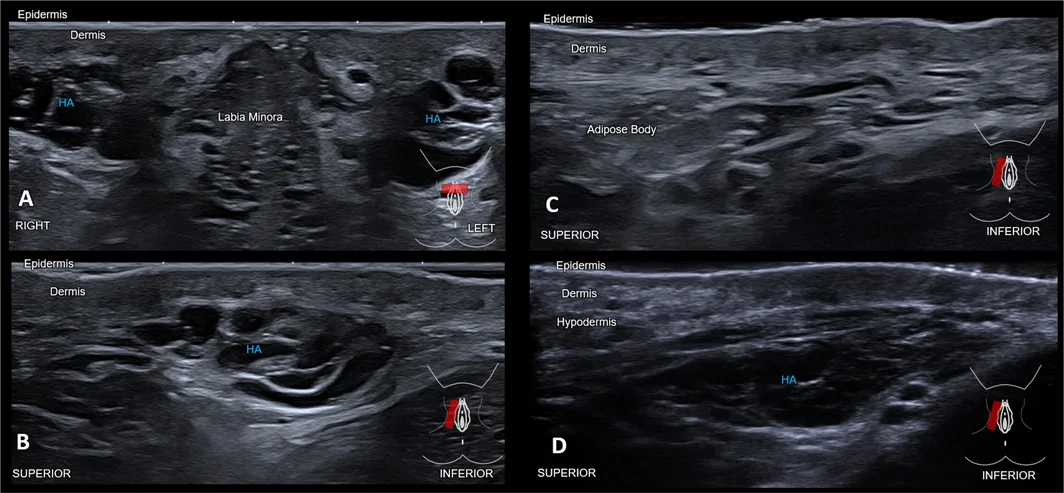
High‐frequency ultrasound (HFUS) images of hyaluronic acid (HA) filler in the labia majora. B‐mode 24 MHz images obtained immediately after injection (A, B) show well‐defined anechoic lobulated HA deposits located beneath the superficial fascia. Images obtained approximately 80 days after treatment (C, D) demonstrate similar distribution and homogeneous echogenicity, with preserved delineation of the fascial and subcutaneous planes.
